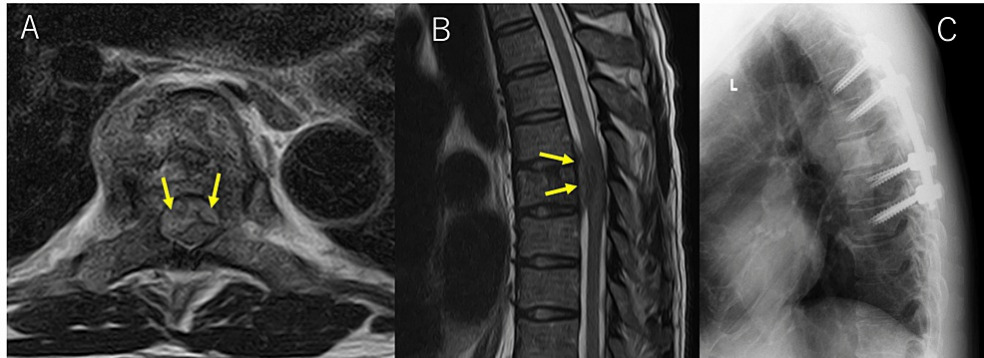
Initial preoperative MRI and postoperative radiograph of the first surgery (A, B) Axial (A) and sagittal (B) T2-weighted MR images were obtained at the Th7 vertebra at admission, showing an epidural mass-causing cord. (C) Lateral radiograph of the thoracic spine postoperatively after the first surgery.
